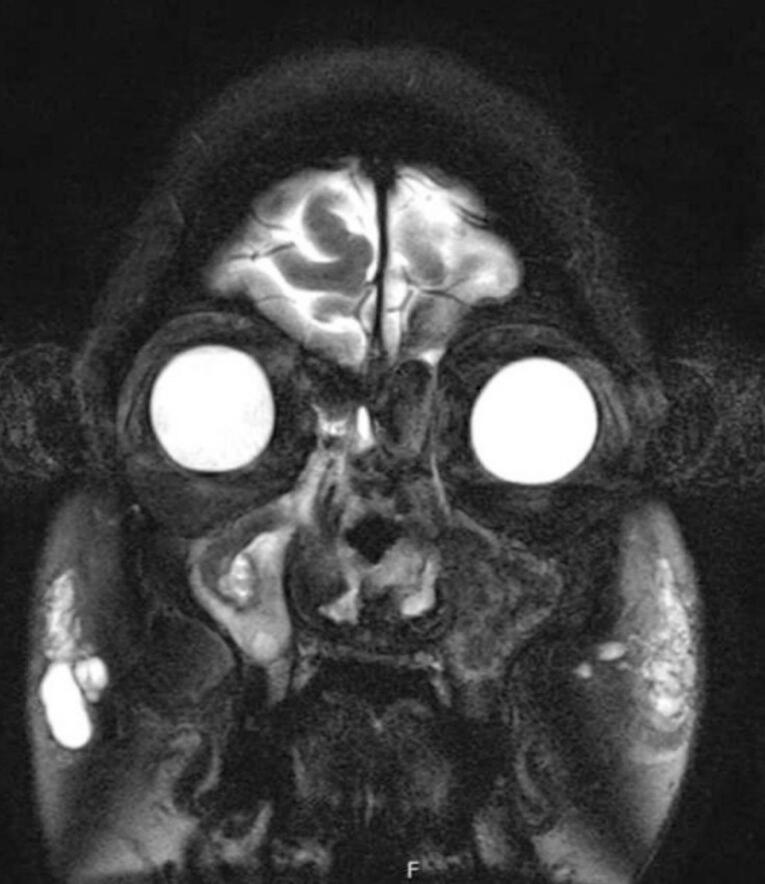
Preoperative coronal T2-weighted MRI of the paranasal sinuses showing an opacification within the bilateral nasal cavities, left maxillary, ethmoid, and sphenoid sinuses.
